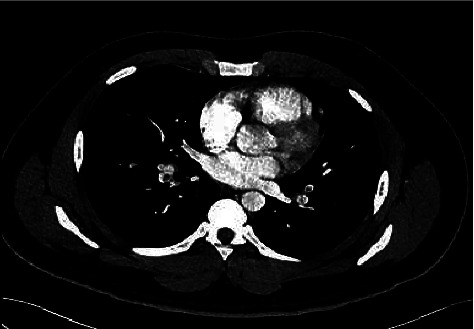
Extensive bilateral pulmonary emboli seen on chest CT angiogram.
